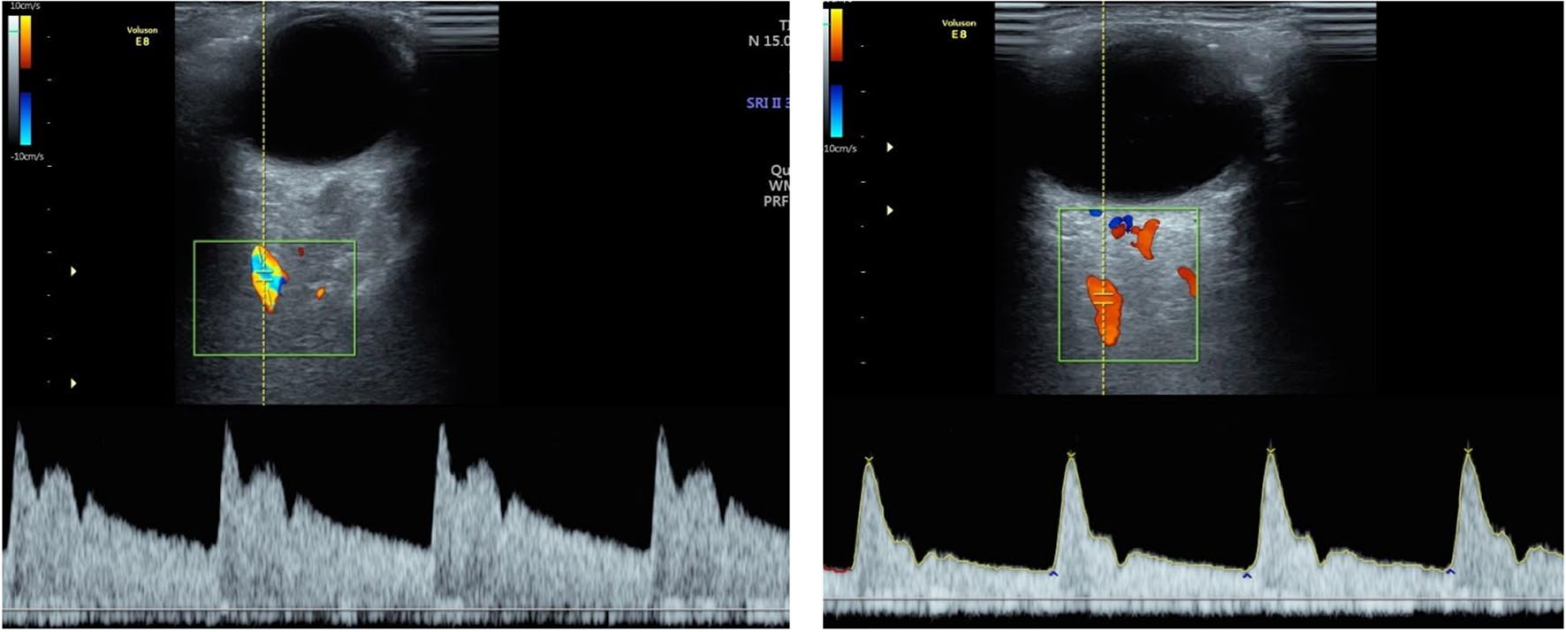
Ophthalmic artery Doppler demonstrating different wave morphology with elevation of the second peak systolic velocity P2 and peak ratio (on the left) compared to Doppler waveform with low P2 and peak ratio in non-smokers group (on the right).
